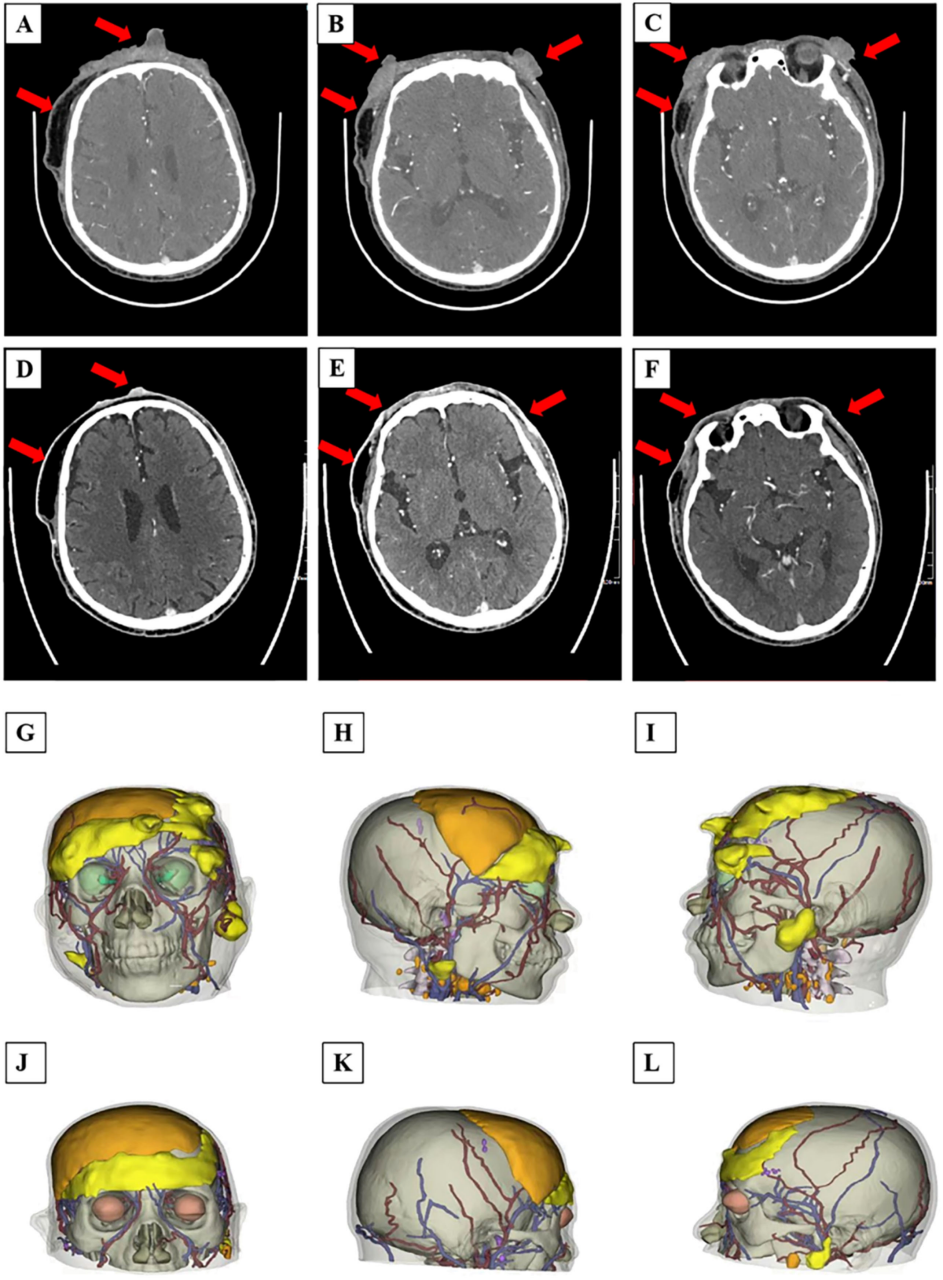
(A–L) CT scan images and 3D reconstruction printing of the patient before and after PDT treatment: (A–C) CT scan presented bilateral periorbital, bilateral frontal, left parietal, right temporal, and left mandibular paracortical subcutaneous soft tissue lesions of the patient after the first time PDT treatment; (D–F) CT scan showed some obvious and significant decrease in size of the recurrent malignant facial lesions before the second time PDT treatment. (G–I) 3D imaging of the patient’s tumor site before treatment, and (J–L) post-treatment. (G, J) the positive view. (H, K) the right view. (I, L) the left view.
